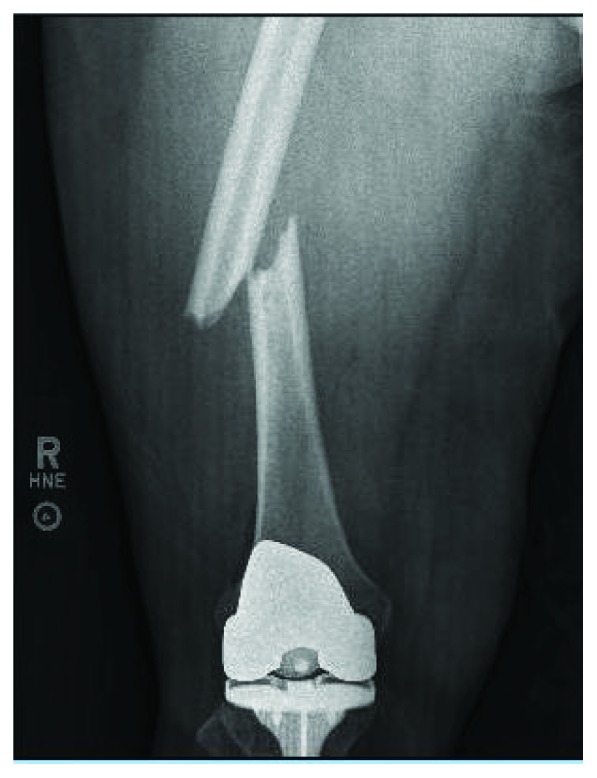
Anteroposterior radiograph of the right femur demonstrating a fracture through femoral diaphysis.
